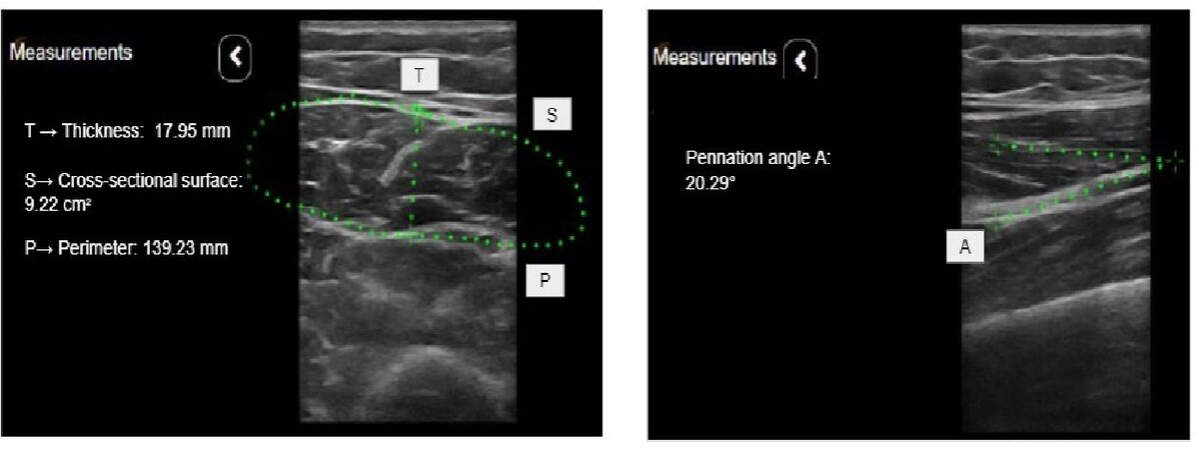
Morphometric ultrasound measurements. (A) Rectus femoris thickness, cross-sectional surface, and perimeter (transverse view). (B) Pennation angle (longitudinal view).
